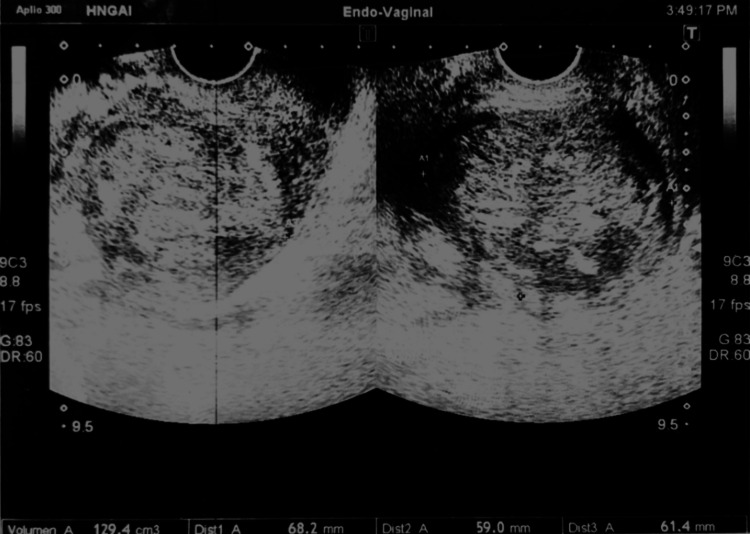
Transvaginal ultrasound: heterogeneous solid tumor, with smooth and well-defined contours, apparently encapsulated, that occupies the uterine body and fundus It measures 68.2 × 59 × 61.4 mm with a volume of 129.4 cc.
